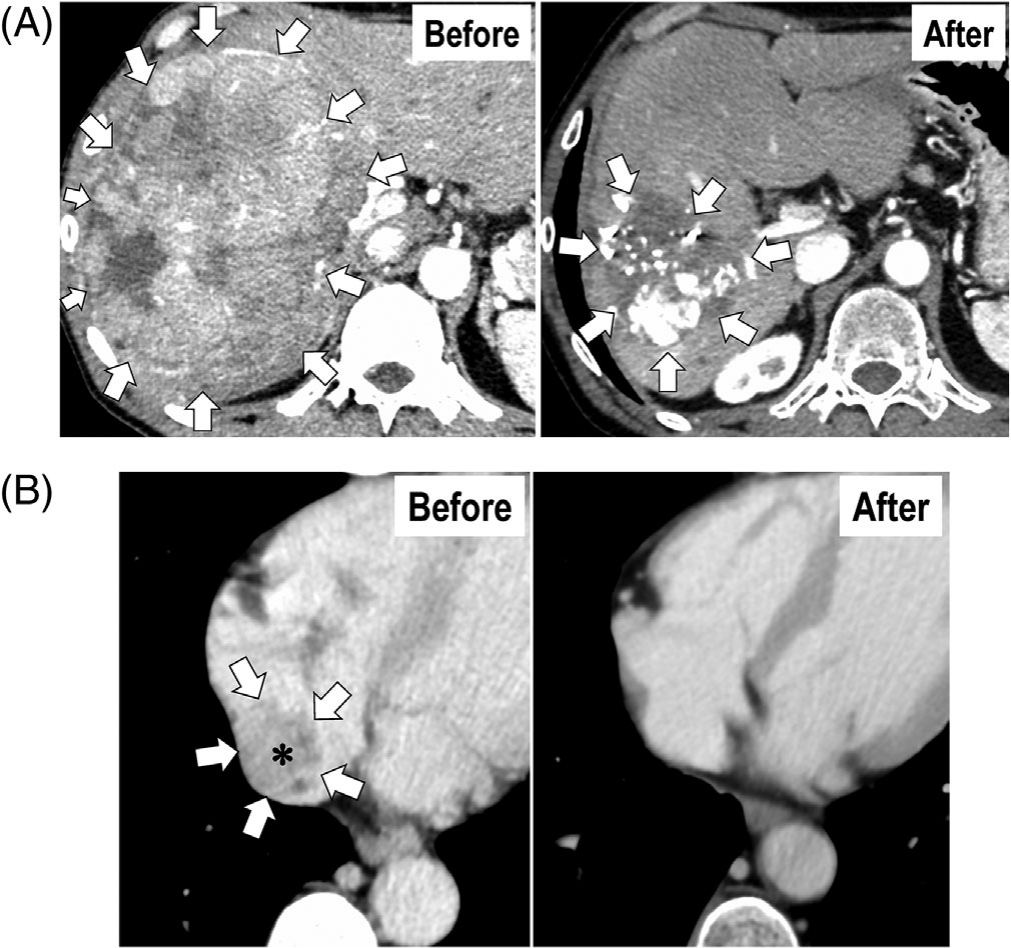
Computed tomography images of a representative patient with unresectable HCC with massive MVI treated with the New FP plus concurrent EBRT. A huge tumor exceeding 20 cm in diameter (A, left image), and massive invasion of the IVC and RA (B, left image) can be seen. White arrows indicate the demarcation of the targeted lesions (A and B). An asterisk (*) demonstrates the tumor thrombus in the RA (B, left image). Following local chemotherapy using the New FP the main tumor in the liver has shrunk remarkably (A, right image), along with the complete disappearance of the tumor thrombus in the RA (B, right image). Before, before the treatment. After, 6 months after the treatment
